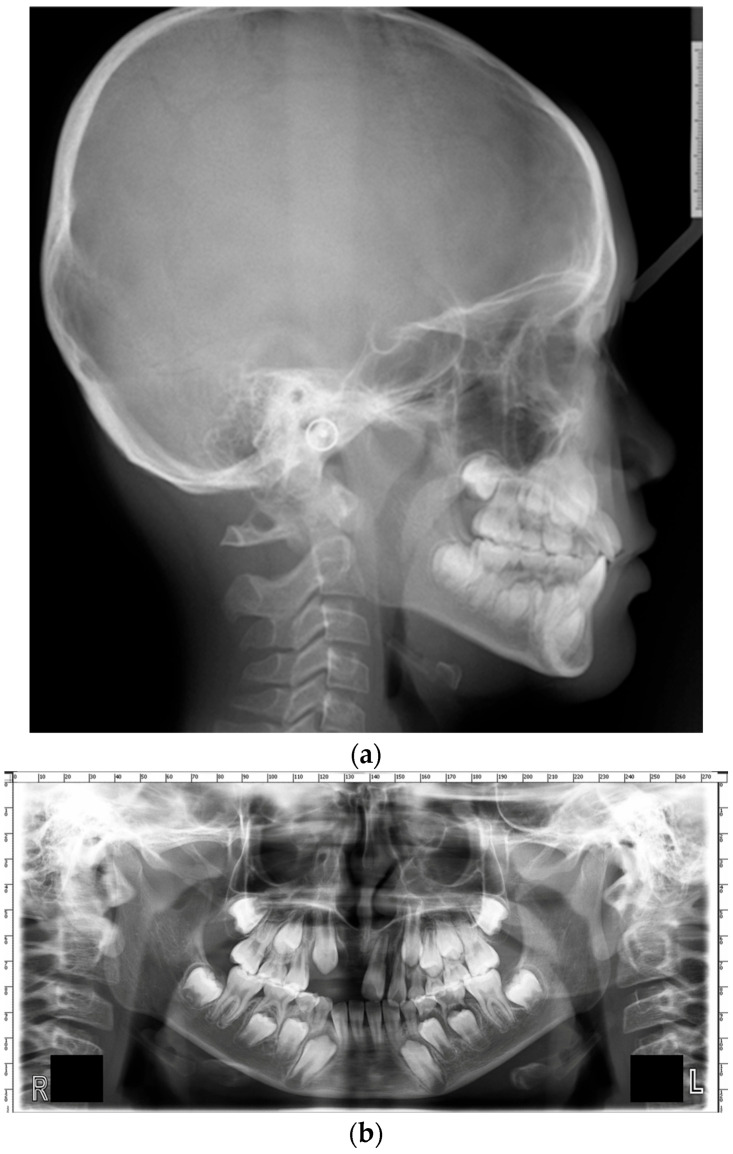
Cephalometric (a) and panoramic (b) X-rays before the initiation of pre-surgical orthodontic treatment, November 2019.
